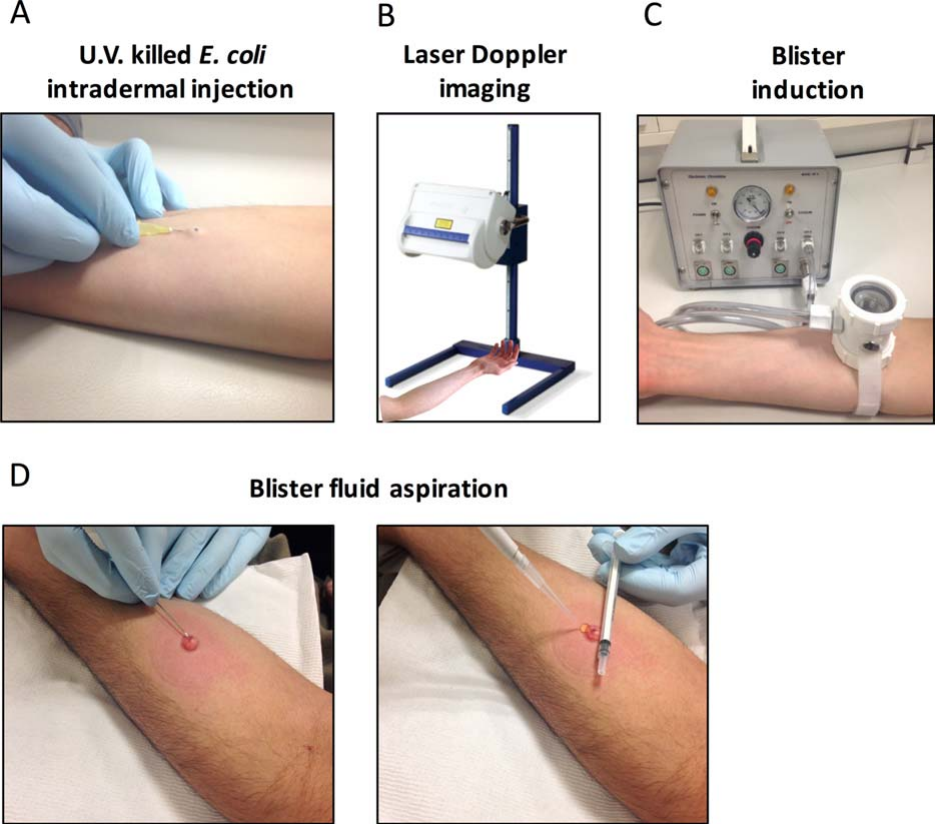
Study protocol. Acute inflammation was triggered in the ventral aspect of the forearm of healthy volunteers by the intradermal injection of 1.5 x 10^7^ UV‐killed *E. coli* (UVKEc) suspended in 100 μl of sterile saline (A). At pre‐defined time‐points after injection, the inflamed site was scanned by a laser Doppler imager to assess blood flow (B). Subsequently, a suction chamber connected to a negative pressure instrument was placed over the injection site to raise a 10 mm blister to acquire the inflammatory exudate present at the site (C). When the blister was completely formed, its roof was pierced using a sterile needle and the contents were aspirated (D).
